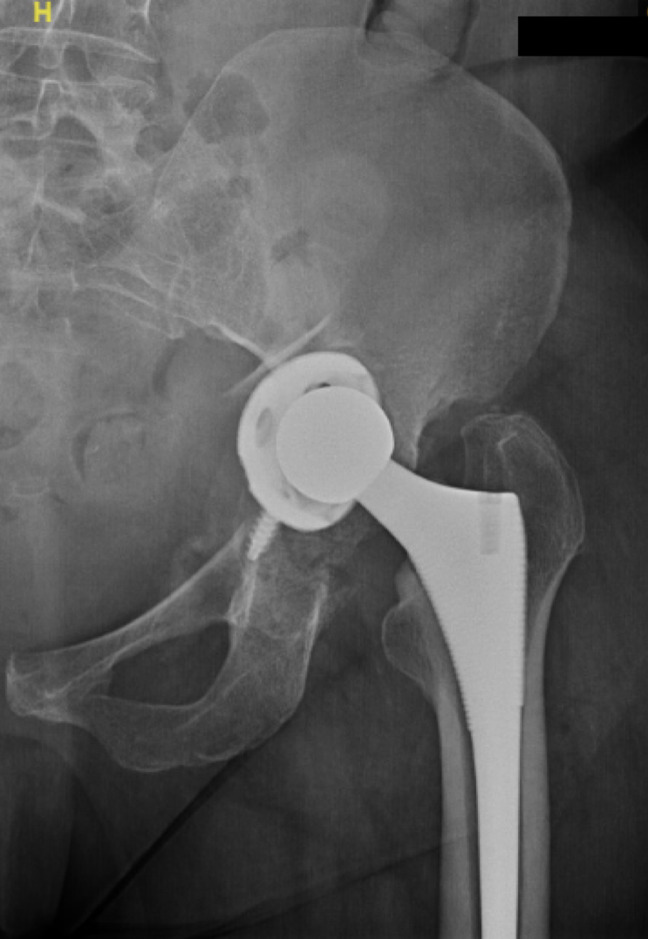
Postoperative radiograph of a patient with acetabular protrusio who underwent total hip arthroplasty complicated by a periprosthetic fracture of the acetabulum.
